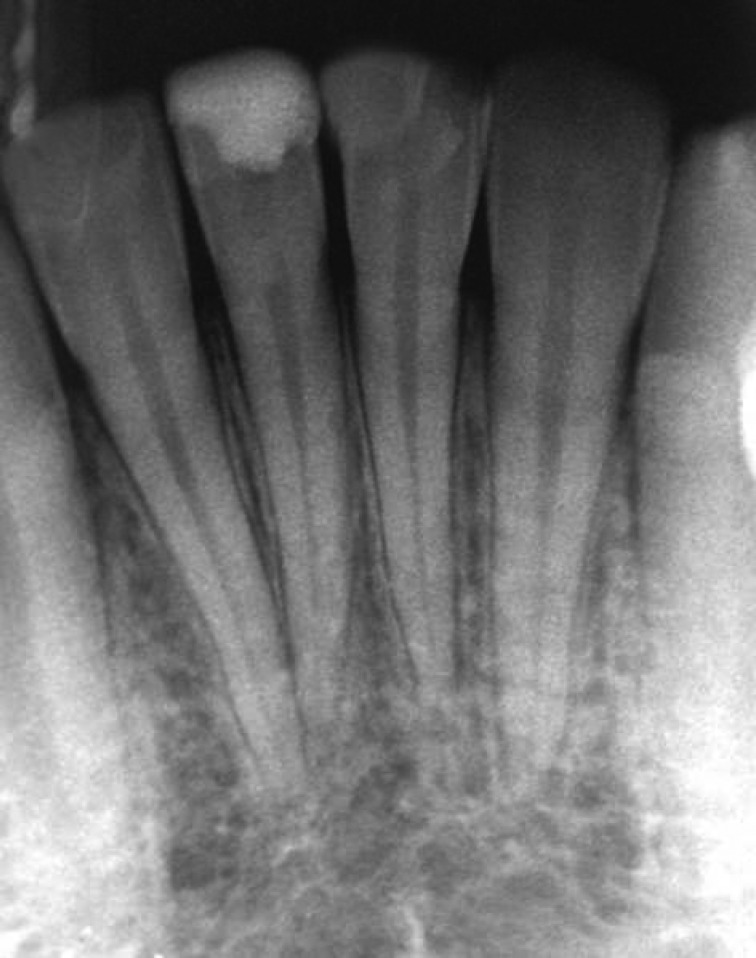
Radiographic image of the teeth 8 years after treatment
